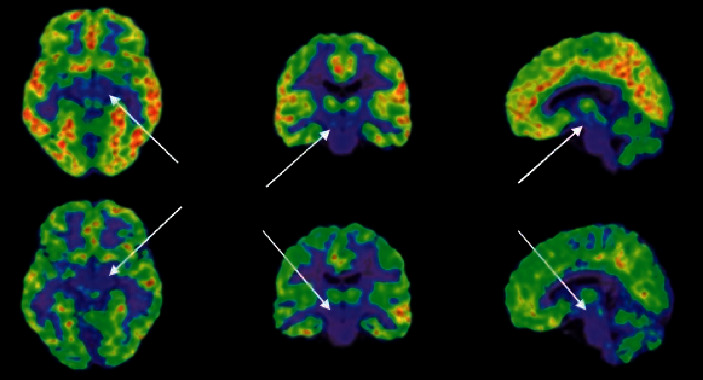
The left striatum of ^18^F-FDG showed slow in and slow out, and the total amount decreased.
